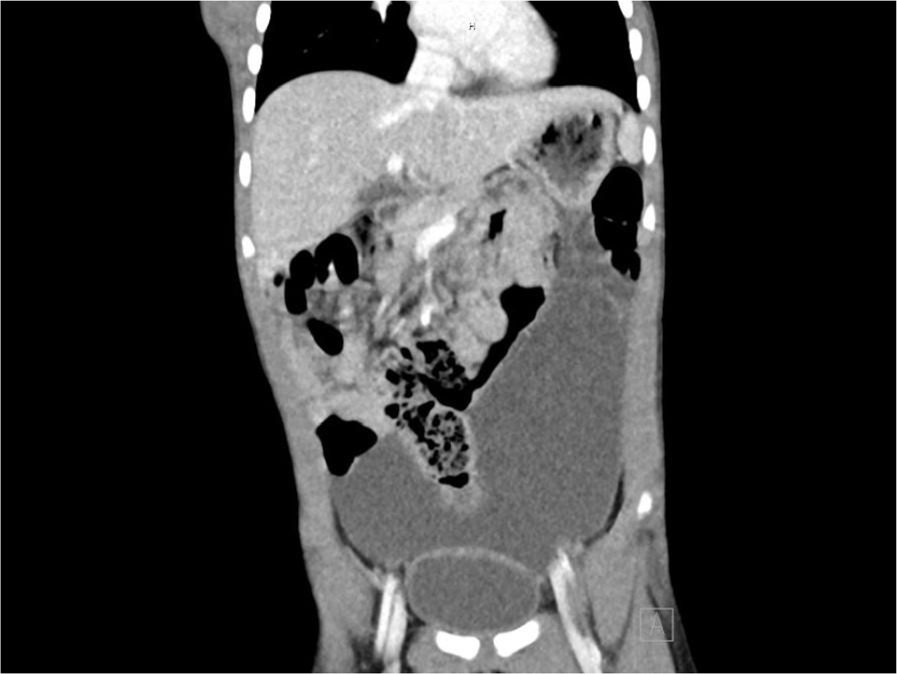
Computed tomography of the abdomen demonstrated a 7.3 × 11.7 × 14.9-cm cystic mass originating from the greater curve of the stomach and reaching the dome of the bladder
